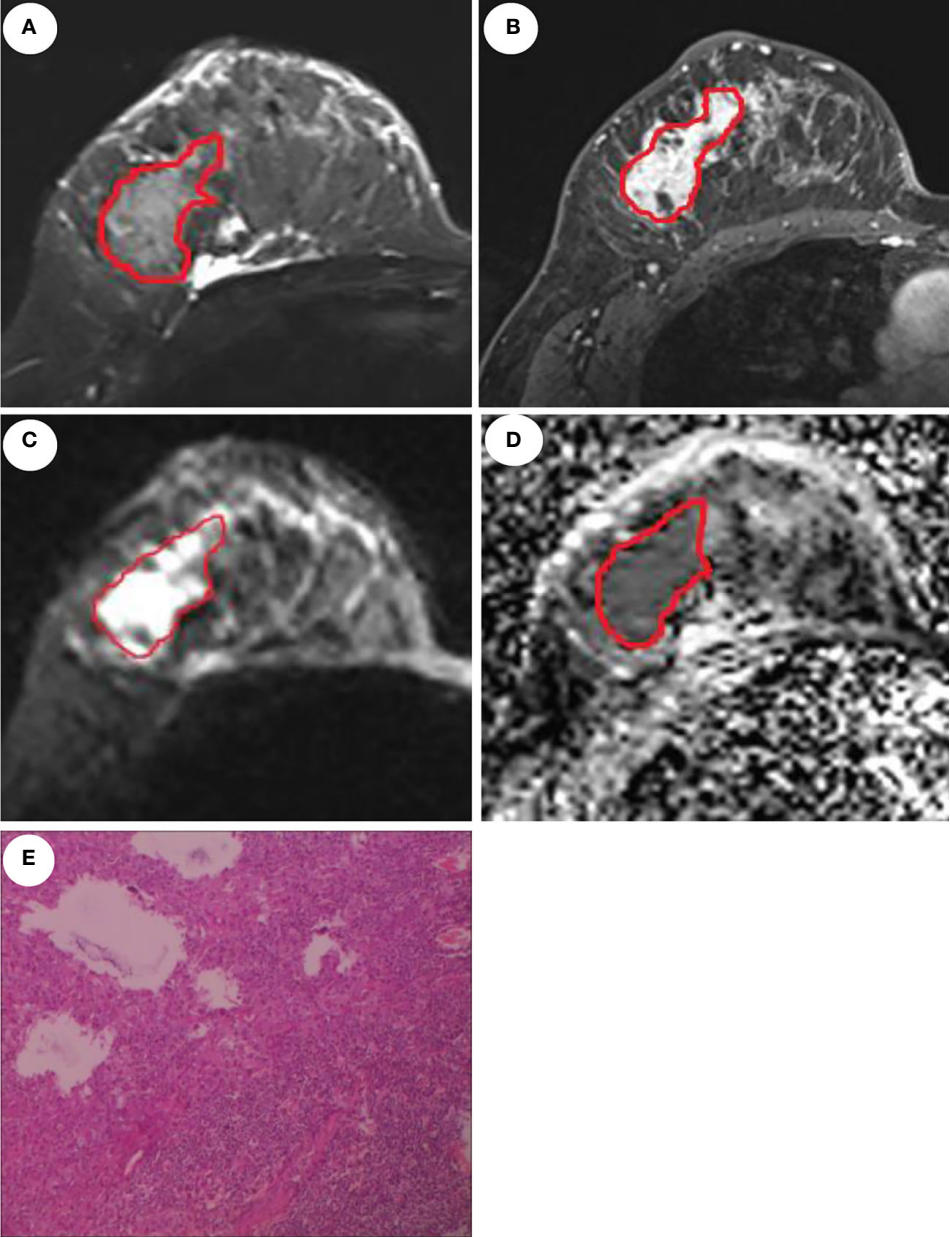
MRI and pathological images of a 46-year-old patient with invasive ductal carcinoma and LVI. Manual delineation of the region of interest on the T2WI (A), DCE (B), DWI (C), and ADC (D), respectively. (E) displays the presence of LVI.
